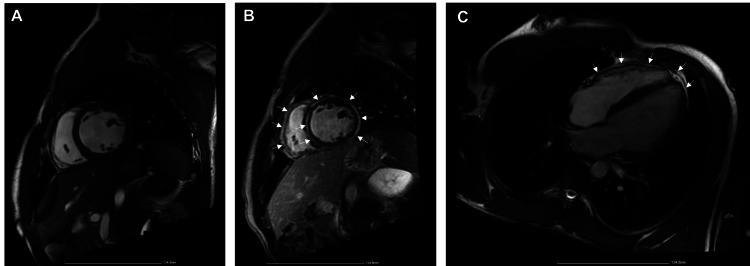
Cardiac magnetic resonance imaging Sagittal plane without contrast (A) showing slightly dilated left ventricle; sagittal plane after contrast injection (B) showing sub-epicardial late enhancement with an annular pattern that progresses through the interventricular septum and extends to the pericardium (C).
